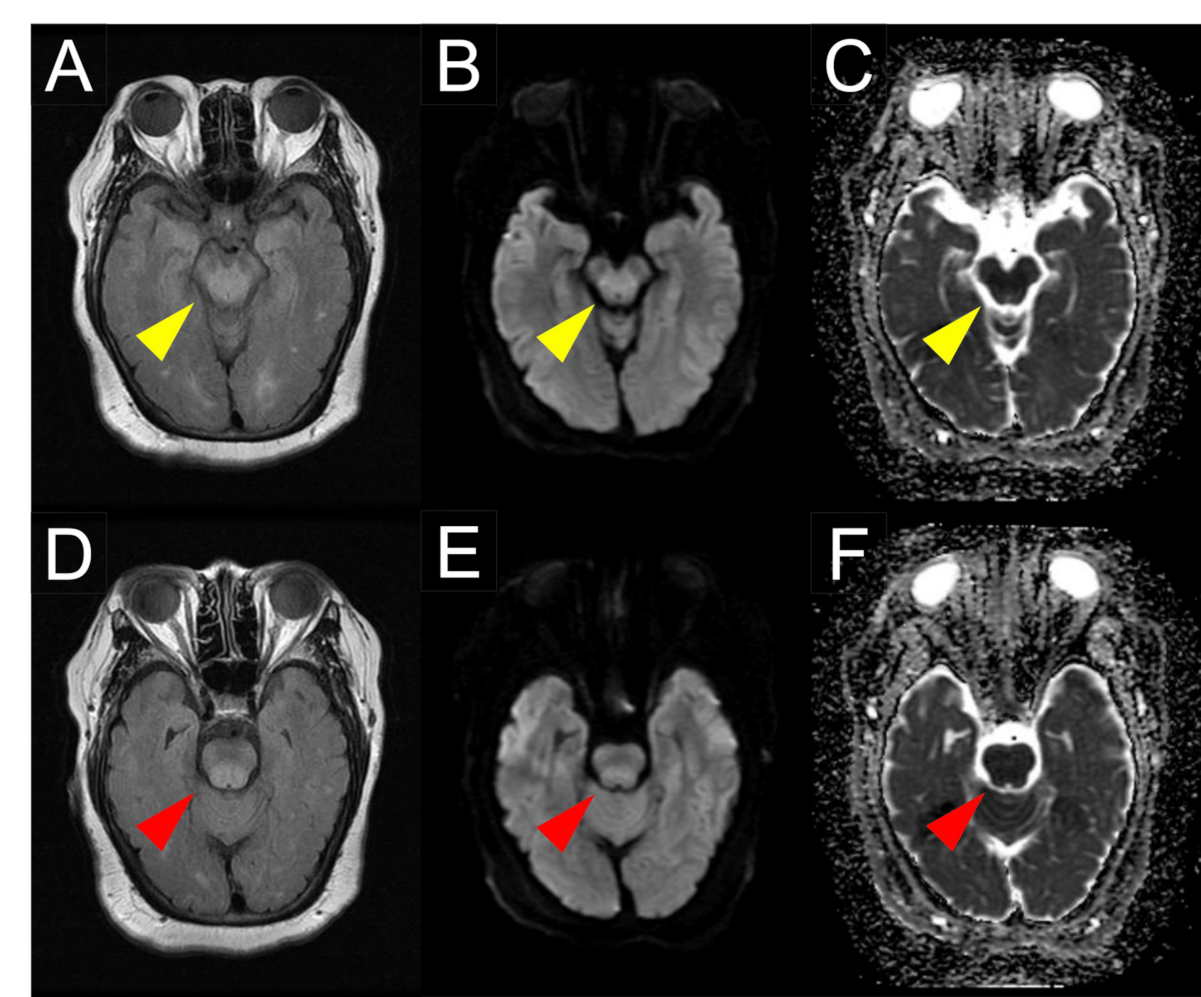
MRI findings on admission MRI showed hyperintensity in the midbrain tegmentum, sparing the cerebral crus, on FLAIR imaging (A), along with hyperintensity on DWI (B) and low values on the ADC map (C) (yellow arrowheads). Additionally, MRI revealed hyperintensity in the pontine tegmentum on FLAIR (D), with corresponding hyperintensity on DWI (E) and low values on the ADC map (F) (red arrowheads). ADC: Apparent diffusion coefficient; DWI: Diffusion-weighted imaging; FLAIR: Fluid-attenuated inversion recovery
